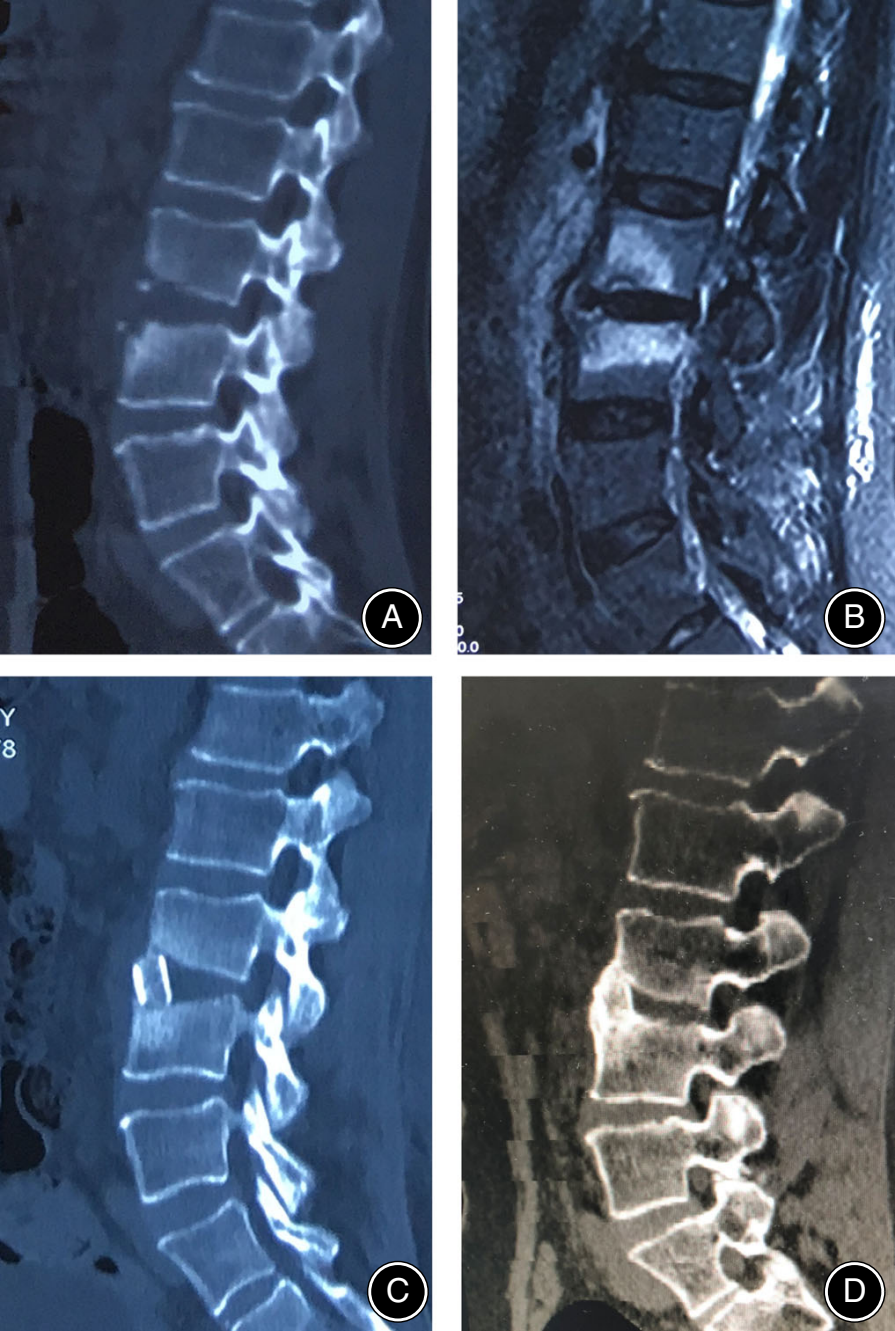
Preoperative CT (A) and MRI (B) images revealed L3/4 intravertebral space infection with superior endplate destruction. A massive structure iliac graft was seen in CT scan (C) before discharge. One year after Mis‐OLIF, CT scan (D) showed bony fusion between bone graft and vertebrae interface.
